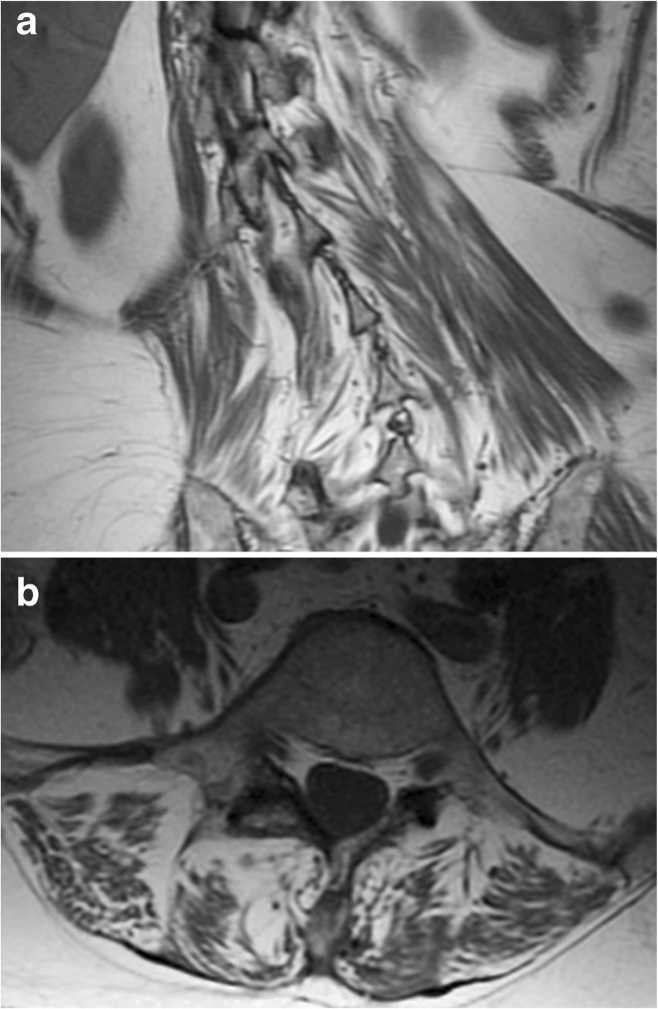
Paraspinal muscle imaging. Fatty replacement of the patient’s paraspinal musculature is demonstrated on coronal T1-weighted MRI image (a), which also shows severe scoliosis, and on axial T2-weighted MRI image at spinal level L4 (b).
